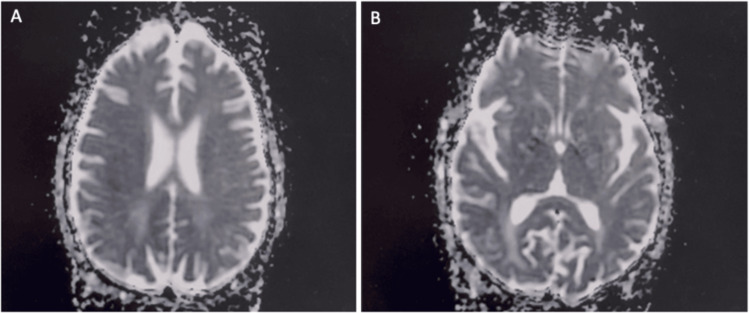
Follow-up brain MRI demonstrating interval improvement. Follow-up MRI of the brain demonstrates marked improvement of previously noted signal abnormalities within the supratentorial compartment, with minimal residual gliosis in the posterior cortices. (A) Axial image showing near-resolution of prior posterior hyperintensities. (B) Axial image confirming significant interval improvement with minimal residual signal abnormality.
